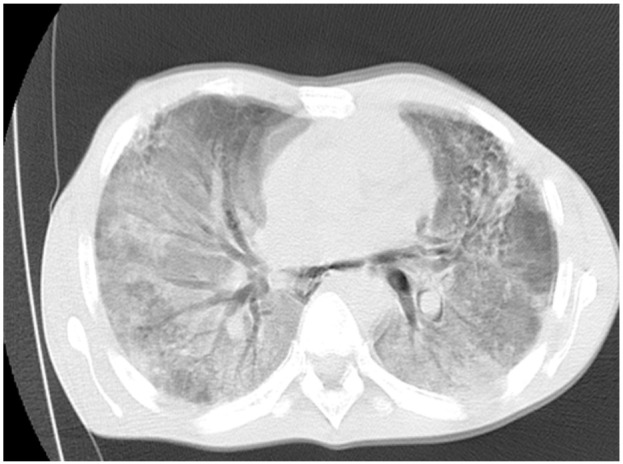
HRCT, lung window: worsening bilateral ground glass opacification with associated reticulations
